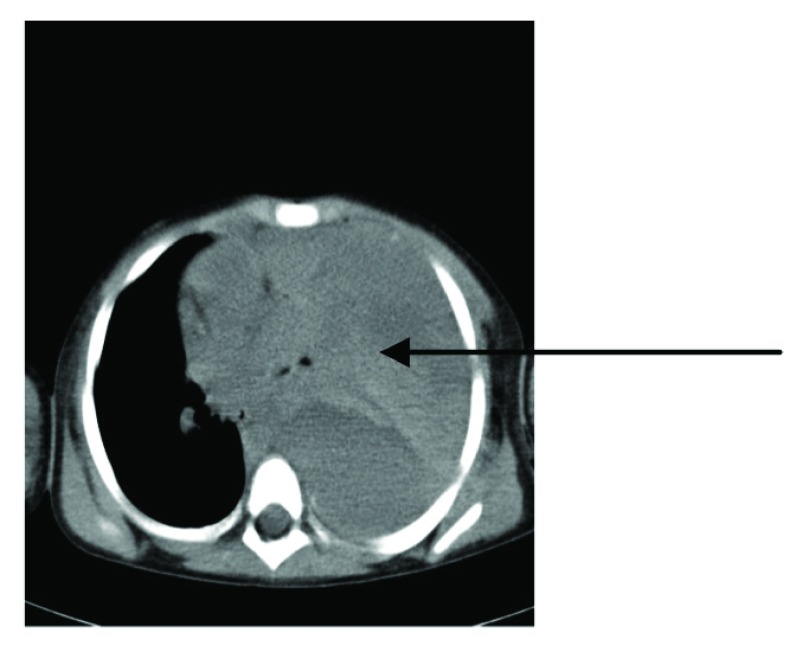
CT scan of thorax recorded on the 15th day of disease: massive left-sided hemothorax with atelectatic lung (arrow) that eventually required surgical treatment, as well as a careful strategy of protective invasive mechanical ventilation.
